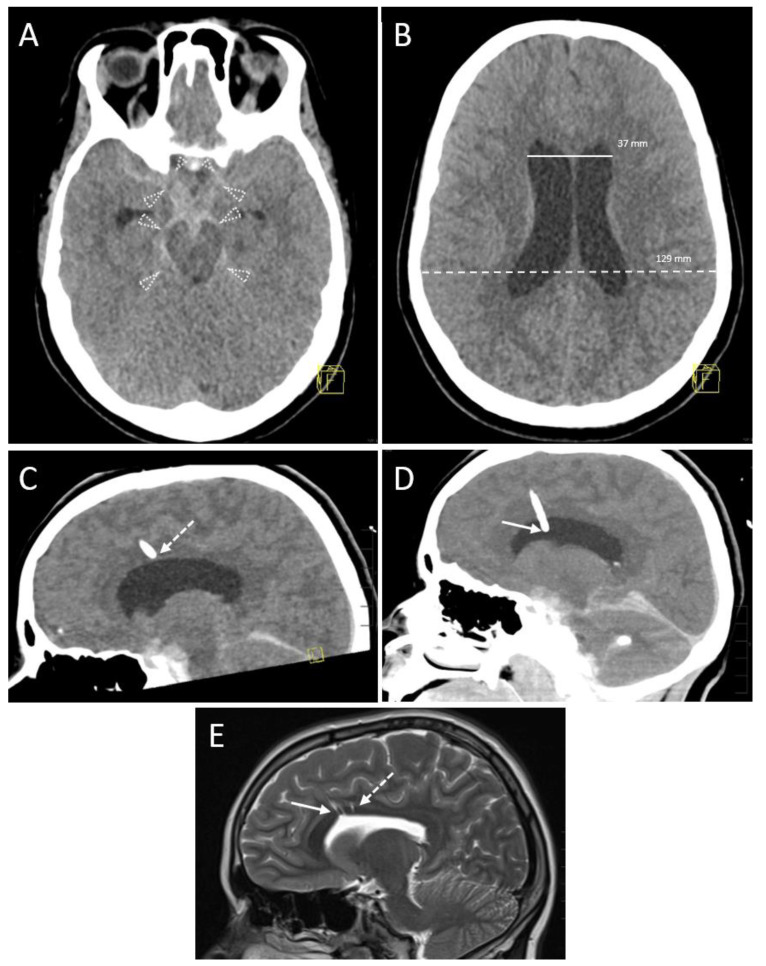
A 14-year-old female with a subarachnoid hemorrhage (Fisher Grade IV) after a motor vehicle accident. (A) Planning a CT scan revealed blood collections in the pentagonal and perimesencephalic cisterns (arrowheads). Yellow graphic: orientation cube (f: frontal). (B) The Evans index of 0.29 (ratio of the maximum width of the frontal horns of the lateral ventricles (37 mm, solid line) and the maximal internal diameter of the skull (129 mm, dashed line)) was in borderline range, suspicious for slightly increased ventricular width. Consequently, the indication for EVD placement was given. Yellow graphic: orientation cube (f: frontal). (C) After EVD placement aspiration of cerebrospinal fluid was not possible. Sagittal reconstruction of the first control scan showed a malposition of the drainage tip in the anterior body of the corpus callosum (dashed arrow). Yellow graphic: orientation cube. (D) Sagittal Maximum Intensity Projection (MIP; 6 mm slice thickness) of the control scan after repositioning of the EVD. The drainage tip is now correctly placed in the anterior horn of the lateral ventricle (solid arrow). (E) A control MRI scan 2 years later shows the puncture channels of the EVD malposition (dashed arrow) as well as their correction placement (solid arrow) as parenchymal defects.
